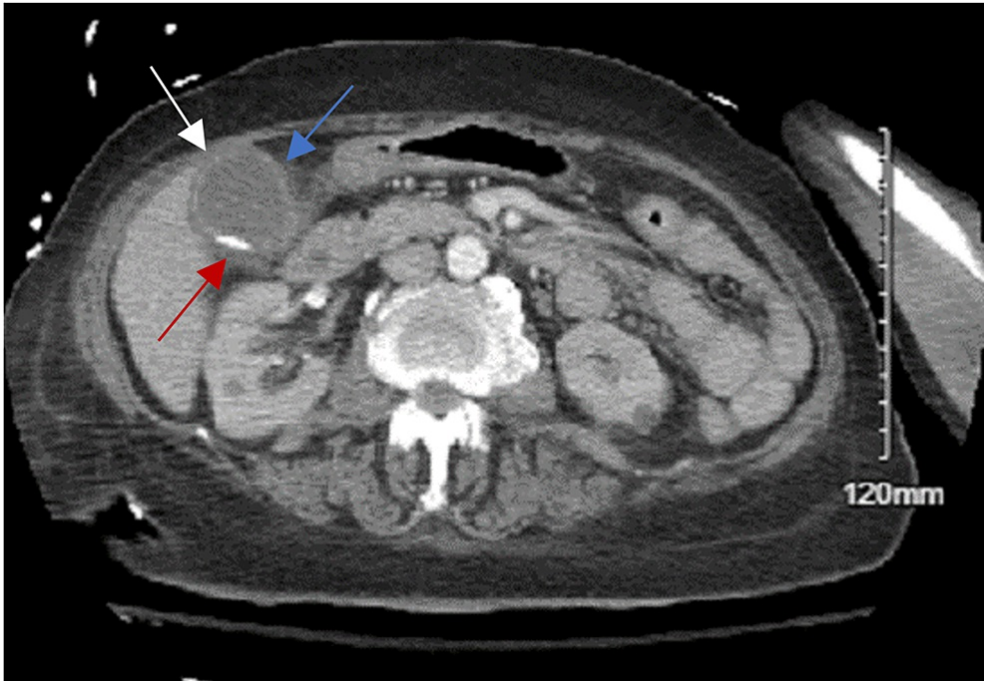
CT abdomen and pelvis with IV contrast. Distended gallbladder (white arrow), cholelithiasis (red arrow) with gallbladder wall thickening (blue arrow), concern for acute cholecystitis. No common bile duct dilatation.
